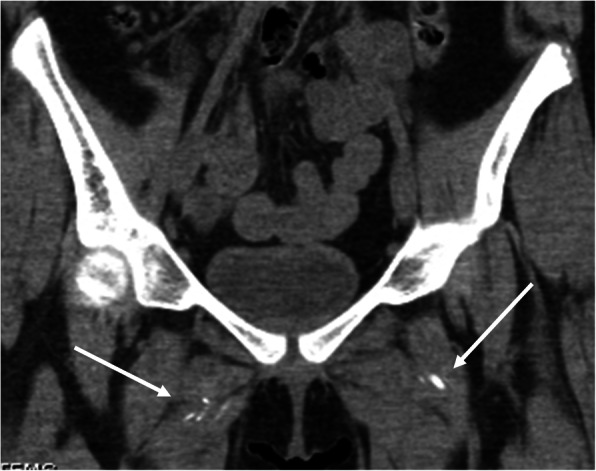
Coronal CT image from the same examination as Fig. 1 shows additional areas of soft tissue calcification in the right and left adductor musculature (white arrows)
